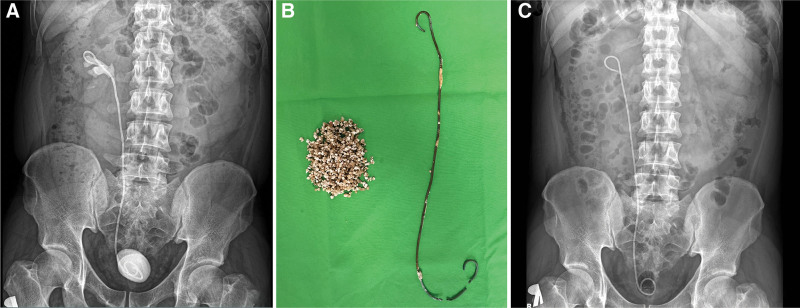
Removal of encrusted DJ stent using a combined endourological approach. (A) KUB radiograph of an encrusted DJ stent. (B) Removed encrusted DJ stent. (C) Postoperative KUB radiograph. DJ, double J; KUB, kidney–ureter–bladder.
